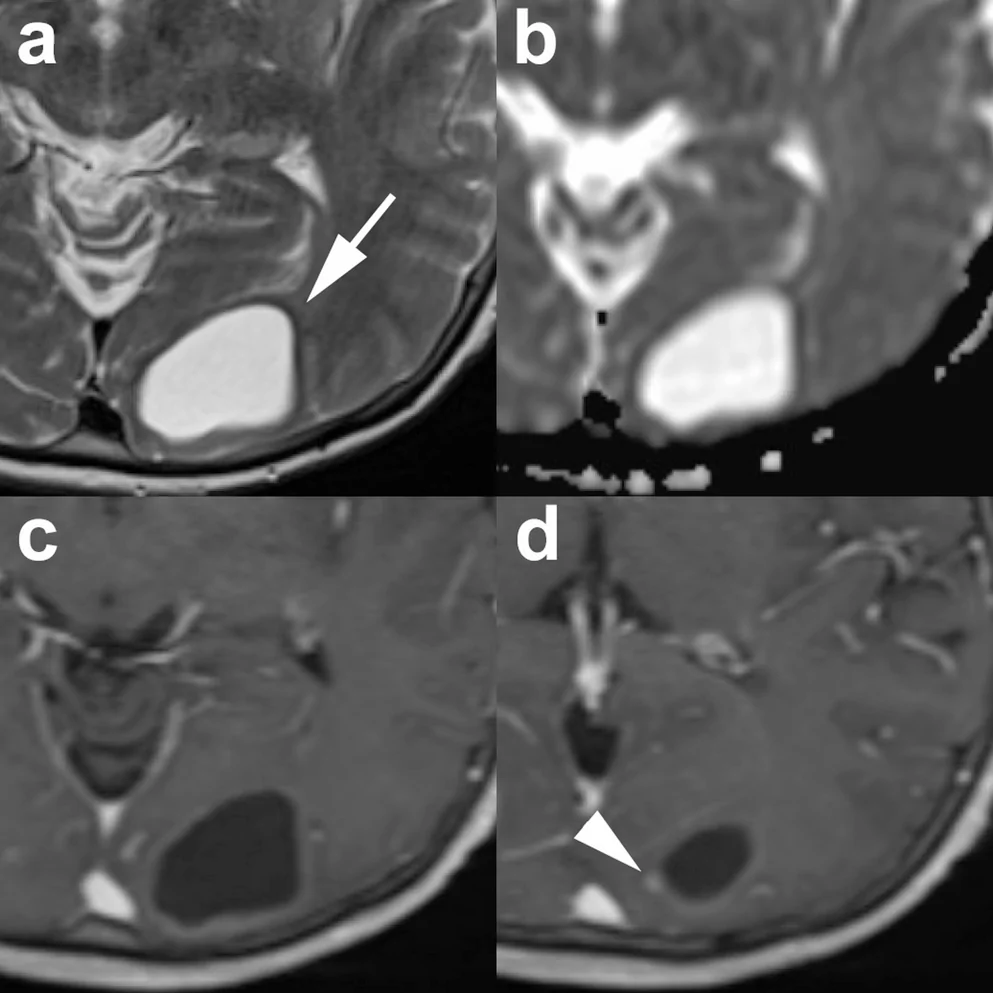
A 69-year-old woman with a history of breast cancer and lung, liver, and bone metastases presented with brain metastases. Axial T2-weighted image (a) demonstrates a homogeneous hyperintense cystic mass in the left occipital lobe (arrow). Contrast-enhanced T1-weighted images (b, c) show slight rim enhancement with a small enhancing mural nodule (arrowhead). The intralesional fluid component demonstrates elevated ADC values (d). Multiple additional lesions with solid and cystic components were present in the brain parenchyma (not shown)
